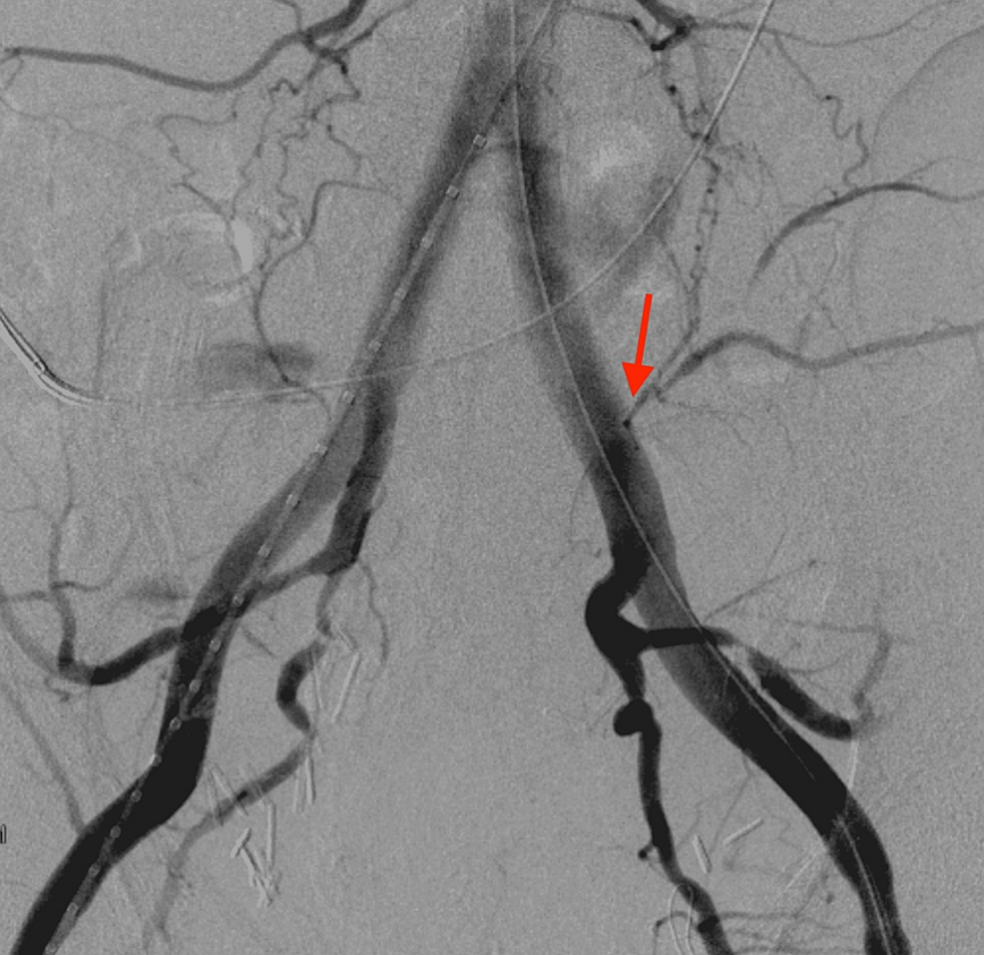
Intraoperative X-ray fluoroscopy. X-ray fluoroscopy revealed extravasation of intravenous contrast from the left common iliac to the ureter.
